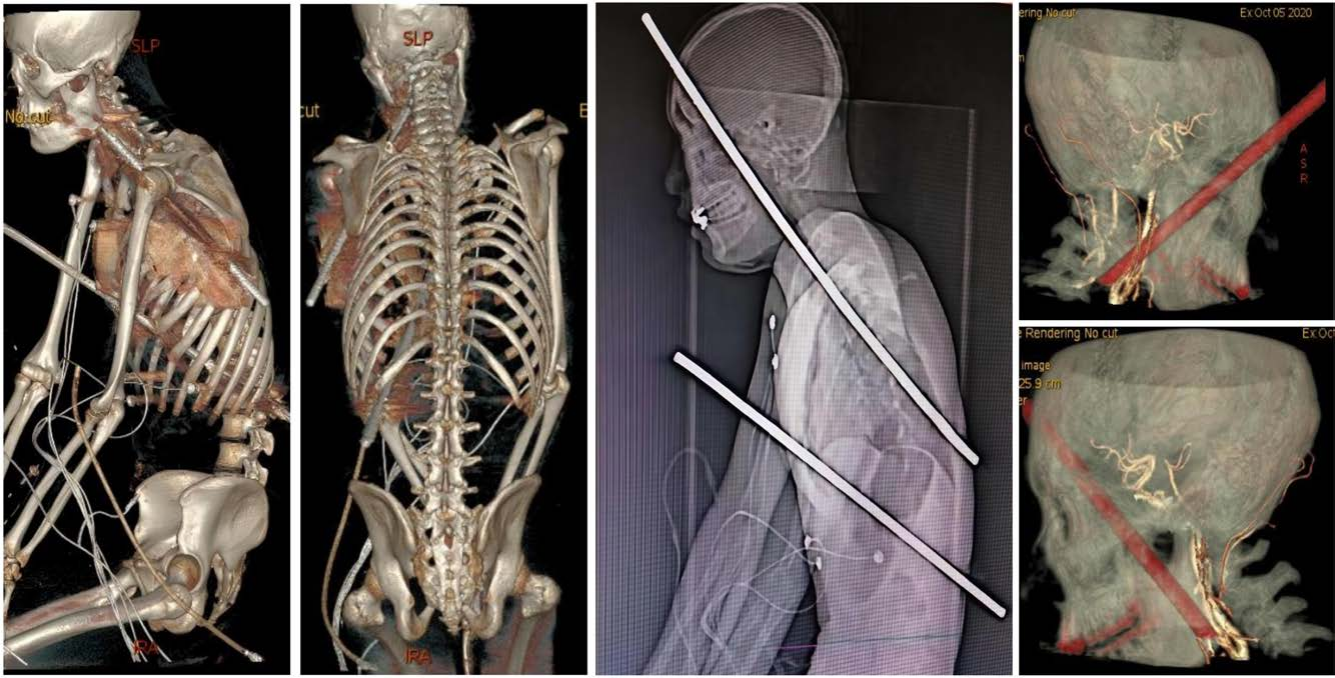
The trajectory of the steel rebar, as confirmed by CT imaging, traversed from the right frontal region, lateral to the right eye socket, into the temporal fossa, through the pterygoid fossa and sphenoid wing, into the oropharynx, along the right cervical vascular sheath, into the left supraclavicular fossa, and into the left axilla. The rebar also penetrated the left fifth costal cartilage, traversing along the greater curvature of the stomach, the splenorenal space, and exiting below the left twelfth rib at the lateral posterior abdominal wall. CT = computed tomography.
